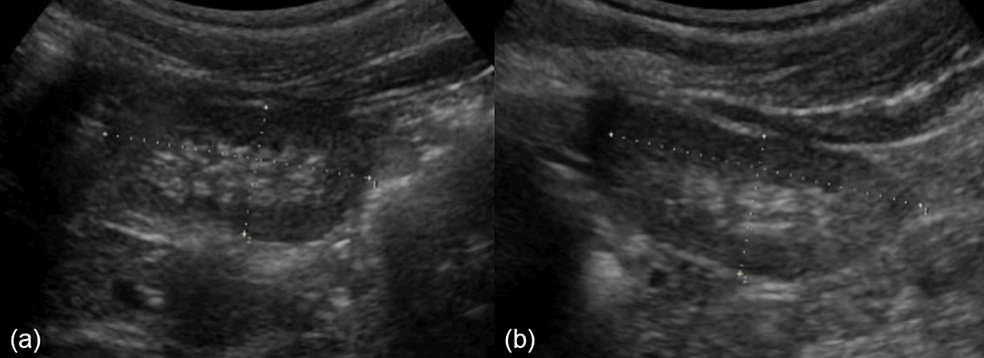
Ultrasonography findings. Increased echogenicity of the renal cortex and bilateral hypoplastic kidney with (a) a right renal length of 5.0 cm and (b) a left renal length of 5.3 cm (-2 standard deviations for her height of 5.7 cm).
